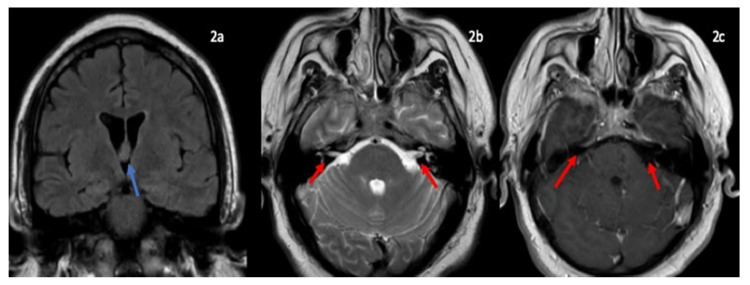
MRI brain coronal FLAIR (2a) showing colloid cyst in the foramen of monro (blue arrow). Axial T2-weighted (2b) axial T1 post contrast images (2c) showing cranial nerve VII and VIII nerve complex (2b) and no abnormal enhancment (2c) (red arrow).
